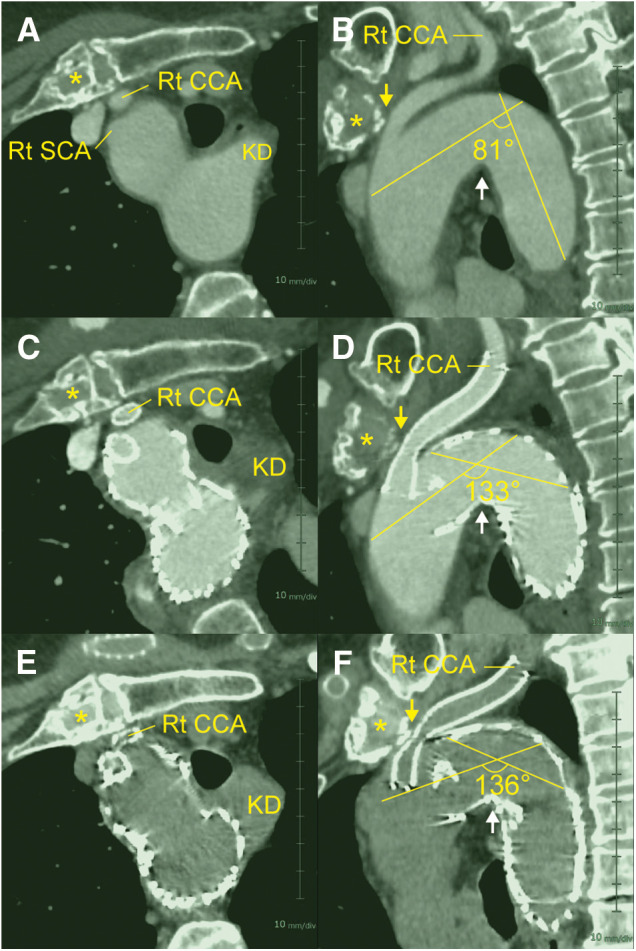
Fig. 3 Morphological changes in the right common carotid artery and aortic arch. The CT scans were taken preoperatively (A and B), prior to discharge (C and D), and 6 months postoperatively (E and F). The most compressed site of the right common carotid artery at each time point was demonstrated axially or sagittally. Lengths between the first right rib (yellow arrow) and the inflection point of the lesser curvature of the aortic arch (white arrow), measured in planes passing parallel to the proximal portion of the right common carotid artery via the protuberant part of the first right rib (white arrow), preoperatively, prior to discharge, and 6 months postoperatively, were 40.0 mm, 35.2 mm, and 35.8 mm, respectively. The angle of the aortic arch was measured using center lines. KD: Kommerell diverticulum; Rt CCA: right common carotid artery; Rt SCA: right subclavian artery
